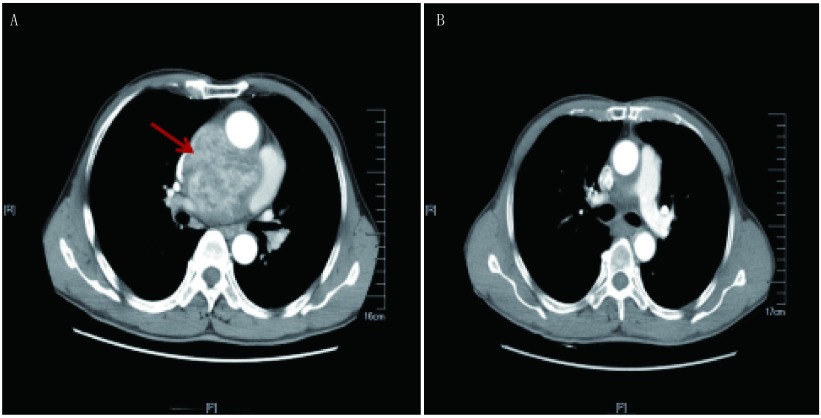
侵袭性纤维瘤术前及术后CT检查。A：侵袭性纤维瘤术前CT检查（箭头示)；B：术后21个月复查CT。 Preoperative and postoperative CT scan of invasive fibroma. A:Preoperative CT scan of invasive fibroma (arrow); B:CT scan of 21 months after operation.
